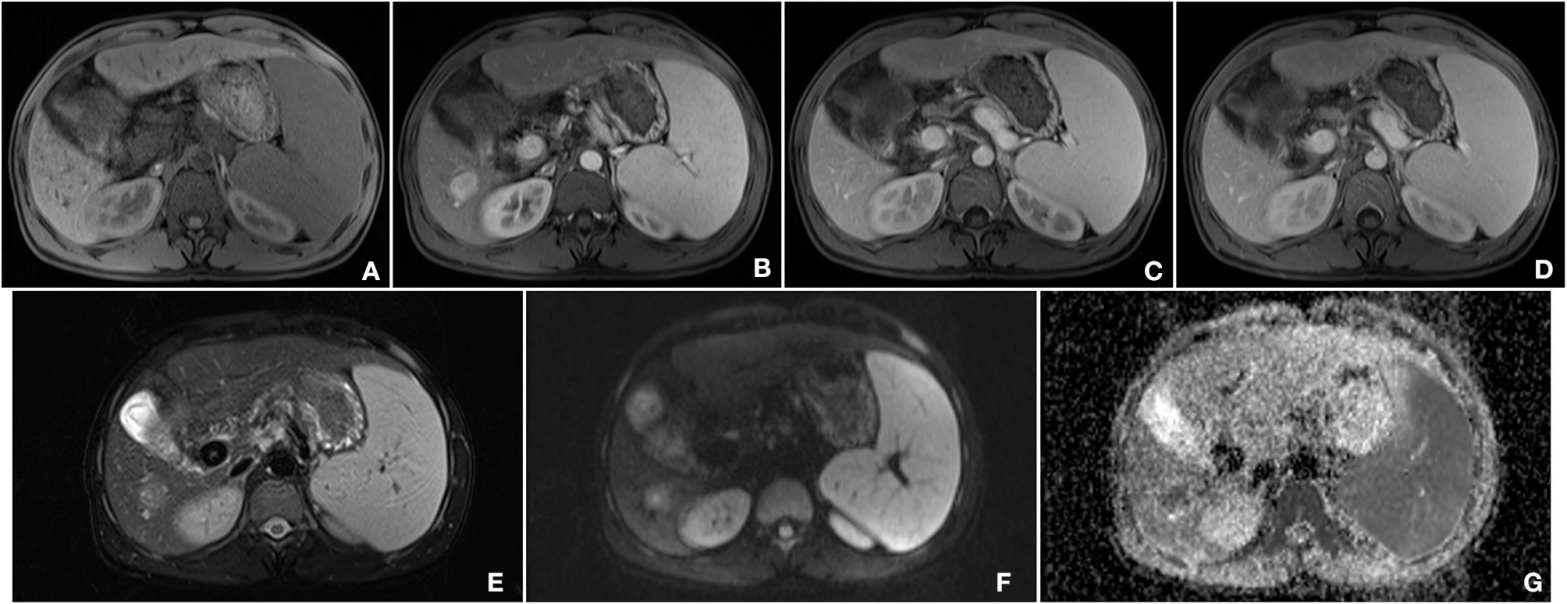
Findings of magnetic resonance imaging (MRI). (A) T1-weighted image of the nodule showed an iso-intensity lesion. (B) In the arterial phase, the lesion was significantly enhanced, and there was wash out both in the portal phase (C) and late phase (D). (E) T2-weighted image of the tumor presented a slightly higher intensity. (F) Diffusion-weighted image of the tumor was a marked hyper-intensity. (G) ADC showed a low-signal lesion.
